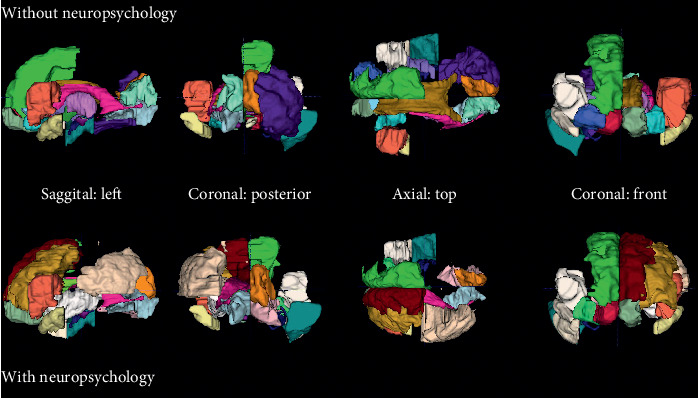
MRI regions selected for prediction of visual-verbal function decline. The following regions were selected for wavelet features: middle and inferior temporal gyrus right, insula right, lateral remainder occipital lobe right, thalamus left, corpus callosum, lateral ventricle excluding temporal horn left, lateral ventricle temporal horn right, third ventricle, anterior orbital gyrus right, bilateral inferior frontal gyrus, superior frontal gyrus right, lingual gyrus left, bilateral cuneus, bilateral medial orbital gyrus, posterior orbital gyrus left, bilateral substantia nigra, bilateral subgenual frontal cortex, bilateral subcallosal area, bilateral pre-subgenual frontal cortex, and bilateral superior temporal gyrus anterior part. For the combination with neuropsychology, the following regions were selected for wavelet features: anterior temporal lobe lateral part right, middle and inferior temporal gyrus right, insula left, middle frontal gyrus left, inferolateral remainder parietal lobe left, caudate nucleus left, lateral ventricle excluding temporal horn left, lateral ventricle temporal horn right, third ventricle, bilateral inferior and superior frontal gyrus, bilateral lingual gyrus, cuneus right, bilateral medial orbital gyrus, posterior orbital gyrus right, substantia nigra left, subgenual frontal cortex right, bilateral subcallosal area, bilateral pre-subgenual frontal cortex, and bilateral superior temporal gyrus anterior part.
